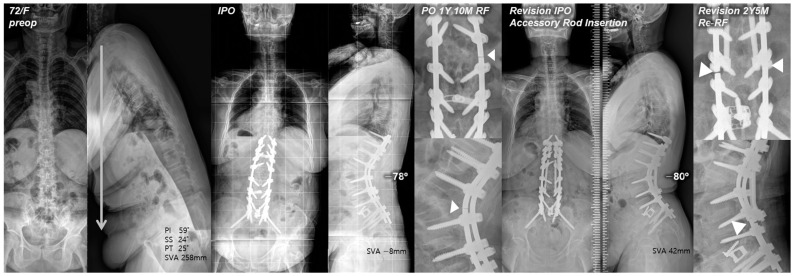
Pre- and postoperative standing radiographs of a 72-year-old female patient. After T10-S1 posterior instrumentation with PSO on L2, and ALIF on L5-S1, optimal sagittal balance was achieved (SVA, −8 mm; TK, 27°; LL, −78°; PI, 60°; PT, 12°; SS, 48°). At 1 year and 10 months after primary deformity correction, RF (right rod) occurred at L2–3. At 2 years and 5 months following revision surgery with bilateral rod replacement and accessory rod insertion, re-RF occurred at L4–5. White triangles indicate sites of RF.
